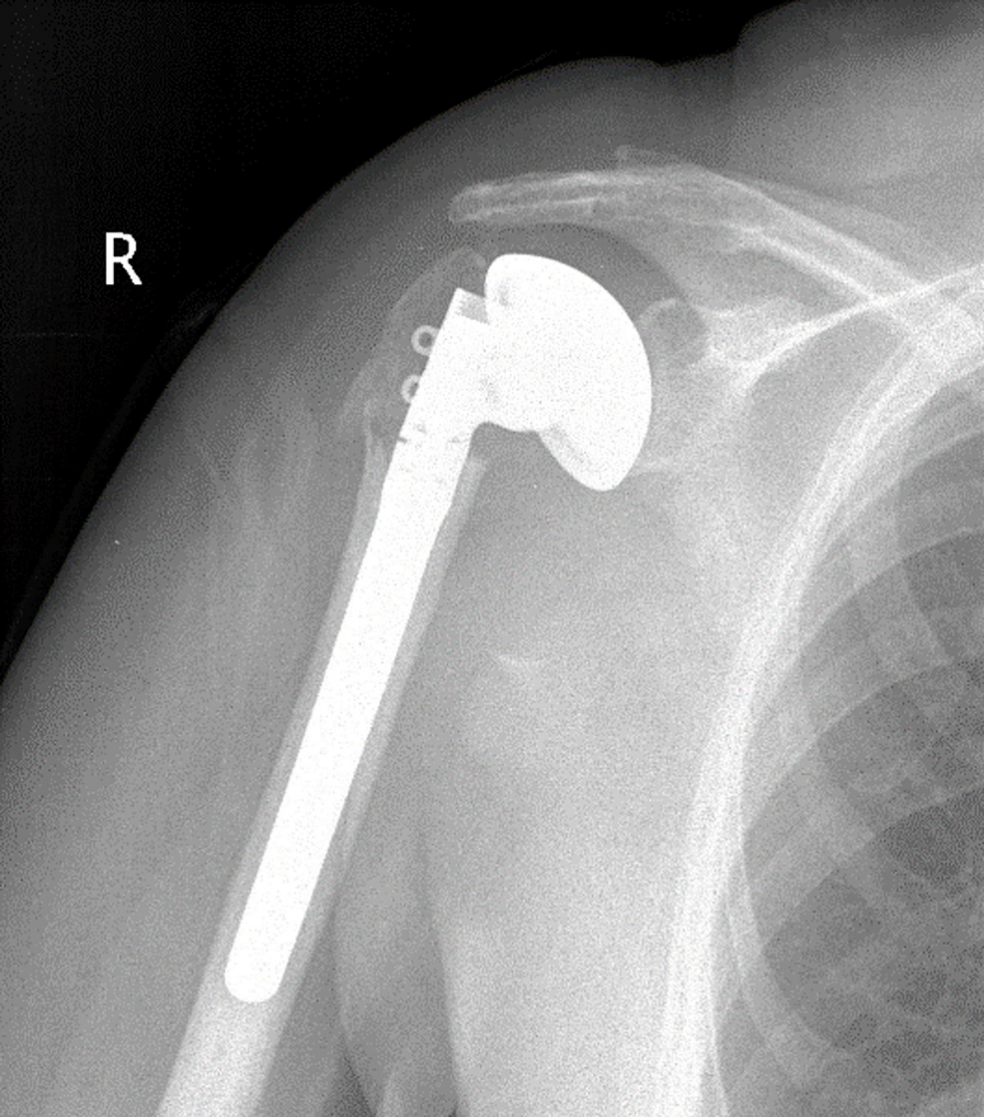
Postoperative X-ray after hemiarthroplasty (HA).
